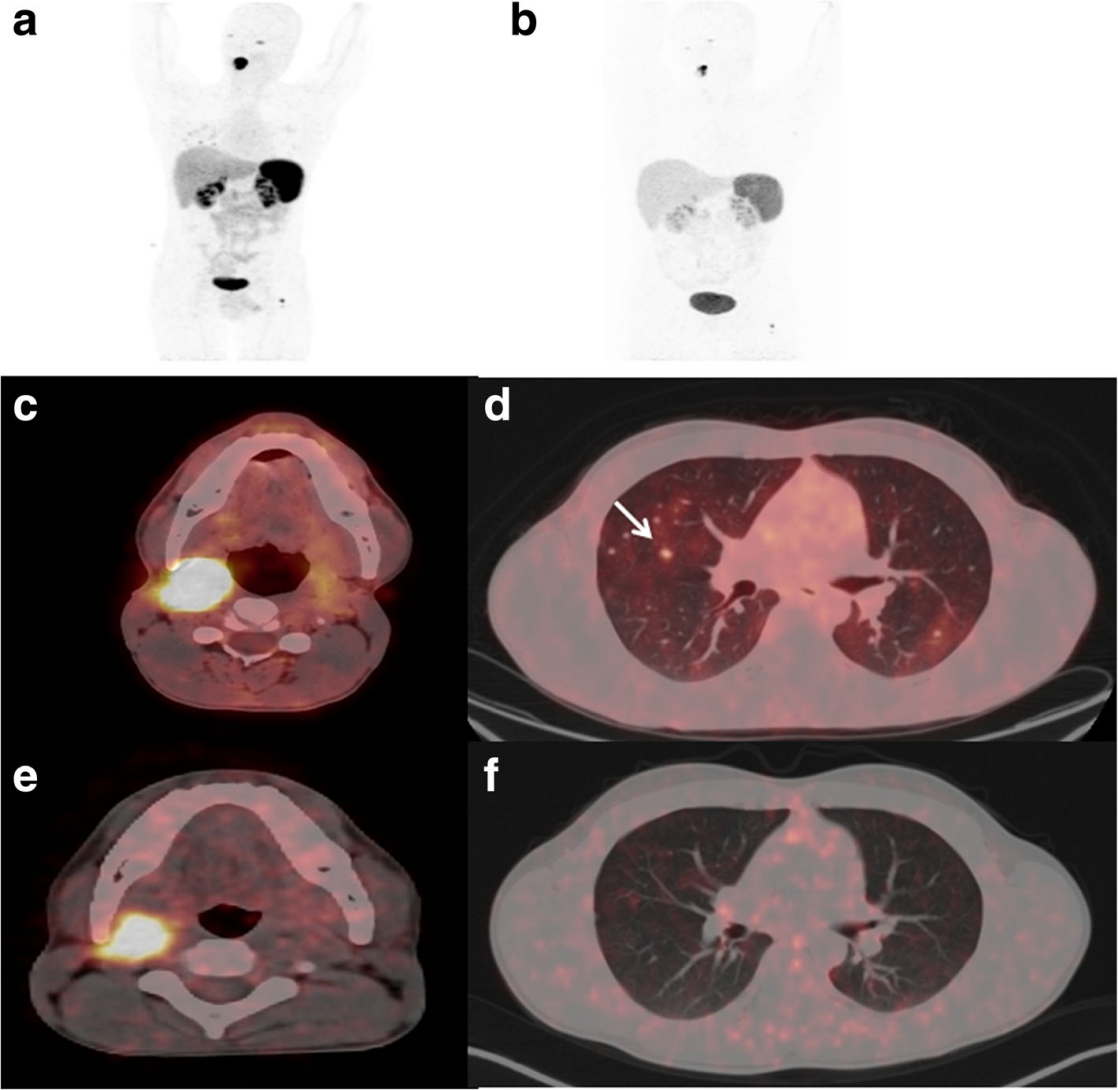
A 22-year-old male was diagnosed with inoperable metastatic right carotid body tumor in 2015. The baseline pre-therapy diagnostic ^68^Ga-DOTANOC PET/CT scan showed somatostatin receptor (SSTR) avid soft tissue mass lesion involving the right carotid body mass measuring 2.6 × 1.7 × 2.7 cm (a, c), with multiple B/L SSTR avid lung nodules (d) and skeletal metastases in the left femur (a). After 4 cycles of ^177^Lu-DOTATATE therapy, the follow-up diagnostic ^68^Ga-DOTANOC PET/CT scan showed partial morphological response with complete resolution of lung metastases and minimal residual disease in the primary tumor (b, e, f)
